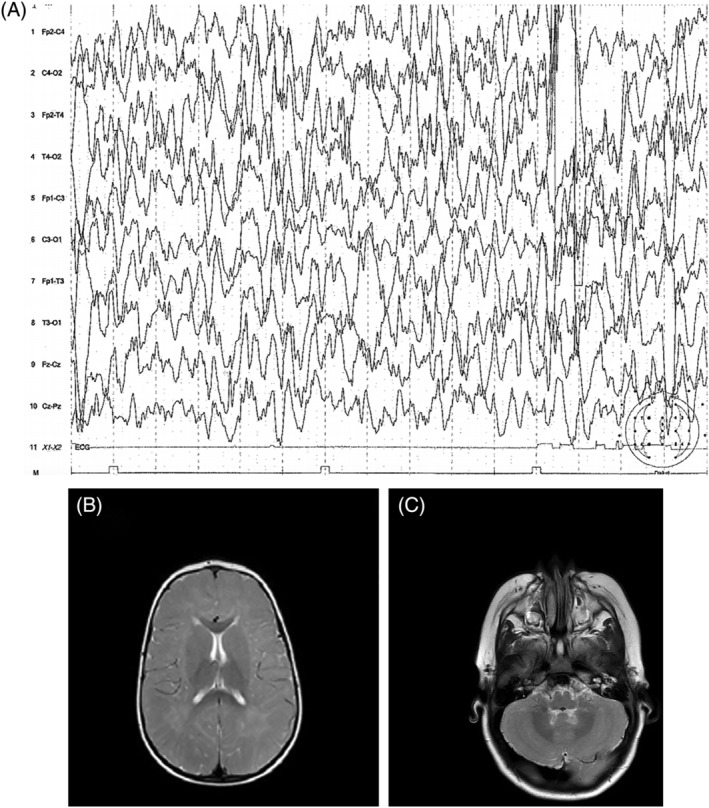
A, Hypsarrhythmic‐like interictal EEG activity of the older sibling at the age of 22 months, marked by theta/delta waves with poor spatiotemporal organization and interrupted by frequent multifocal spikes and intermittent bouts of hypsarrhythmia. B and C, T2 brain MRI of sibling #2 at the age of 18 months, after a few months of per os folinic acid, showing a similar pattern of delayed myelination as his sister, although he clinically presented only mild developmental delay and ataxia
